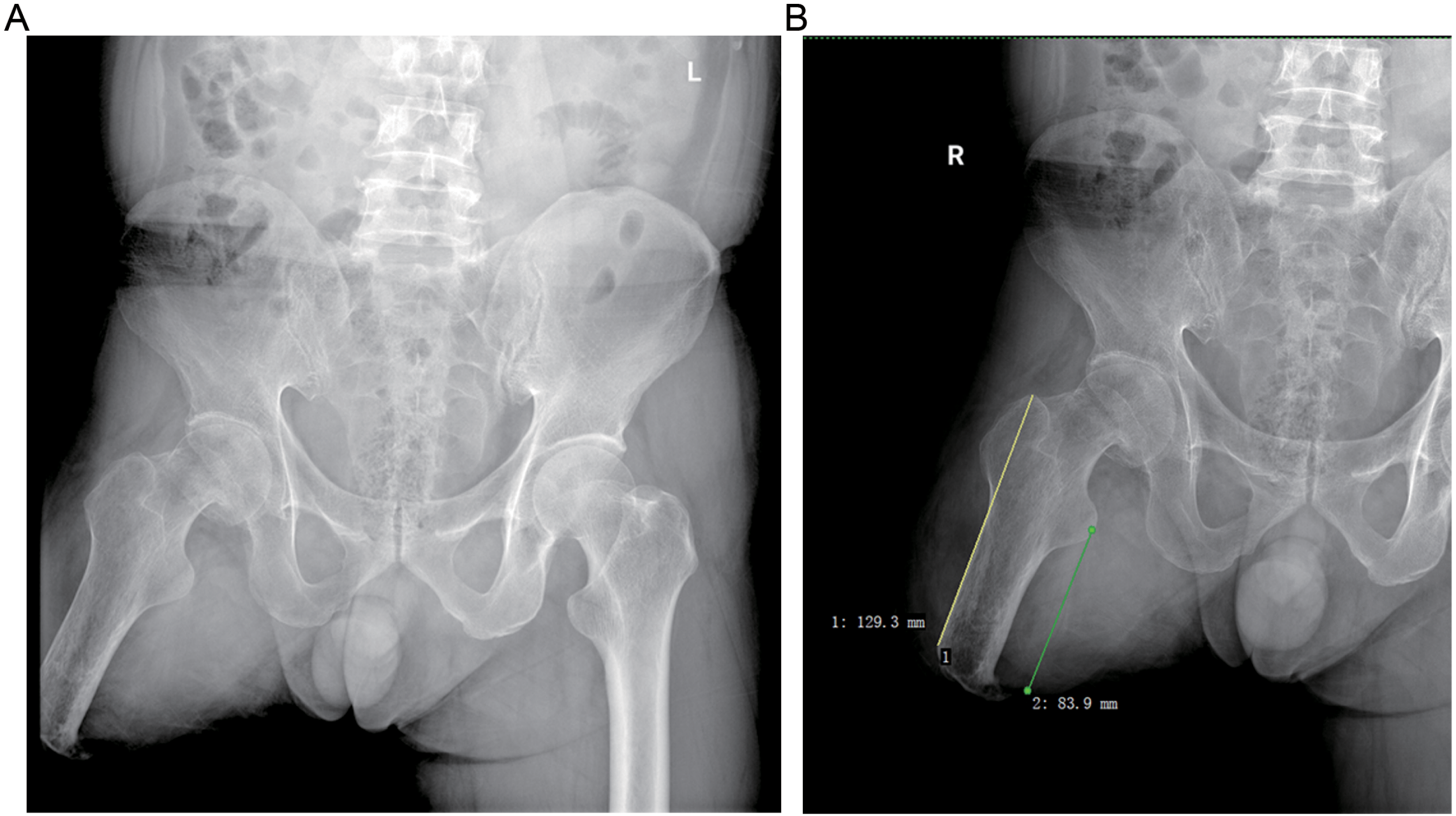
Preoperative radiograph of the patient, showing a femoral neck fracture and signs of bone mass reduction of the right hip. (A) Anteroposterior pelvic x-ray. (B) Anteroposterior x-ray of the right hip joint.
